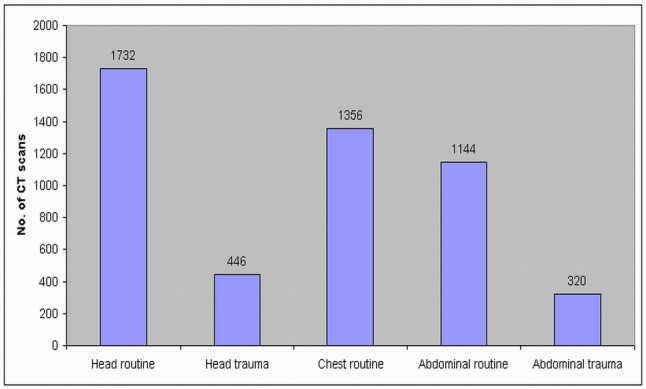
Distribution of the number of CT scans corresponding to different anatomic regions among the three hospitals.
